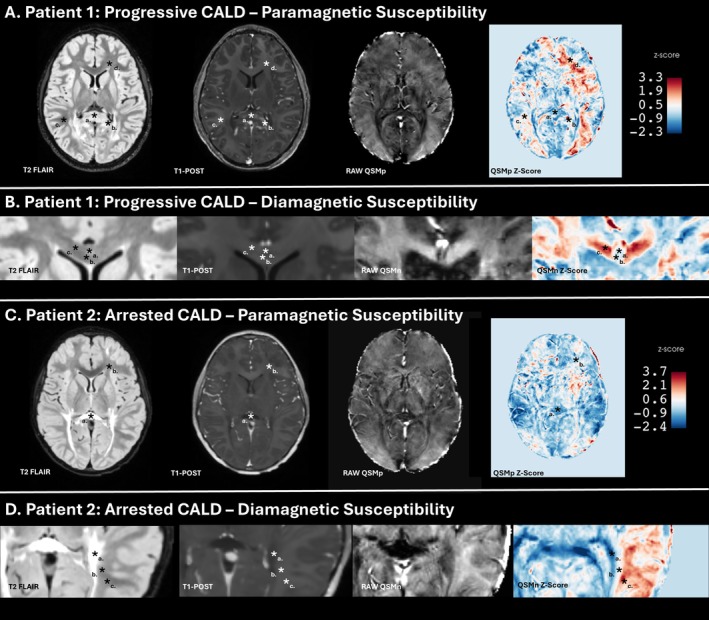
Pathological alterations in iron and myelin signals on source‐separated QSM in CALD. A detailed region of interest analysis across the T2 FLAIR sequence, T1‐post contrast sequence, Raw source‐separated QSM, and QSM *Z*‐score map for Patients 1 and 2. (A) Paramagnetic susceptibility on QSMp in Patient 1 is (a.) decreased in the non‐enhancing area of demyelination, (b.) elevated in the area of enhancement at the leading edge of the lesion, and elevated in (c.) perilesional NAWM and (d.) remote NAWM. (B) Diamagnetic susceptibility on QSMn in Patient 1 is reduced in (a.) corpus callosum lesion and (b.) penumbra of immediate perilesional NAWM, and (c.) increased in distal perilesional NAWM. (C) Paramagnetic susceptibility on QSMp in Patient 2 is decreased in (a.) corpus callosum lesion and (b.) remote NAWM. (D) Diamagnetic susceptibility on QSMn in Patient 2 is (a.) reduced in periventricular white matter lesion, (b.) normalizes in immediate perilesional NAWM, and (c.) increased in distal perilesional NAWM. Corresponding values per coordinate annotation in Figure 1 are provided in Table 1.
